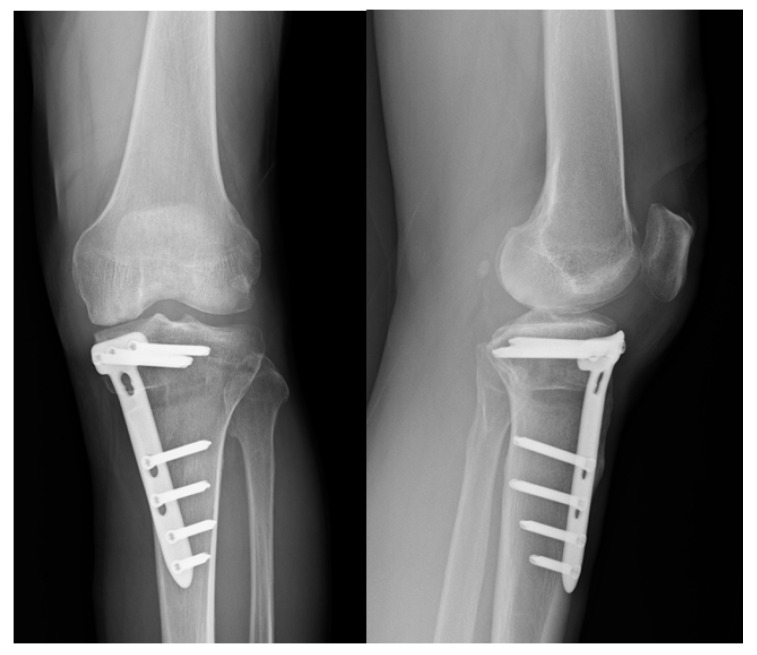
Plain radiographs with anteroposterior and lateral views at the 8-month follow-up showed solid bony union.
